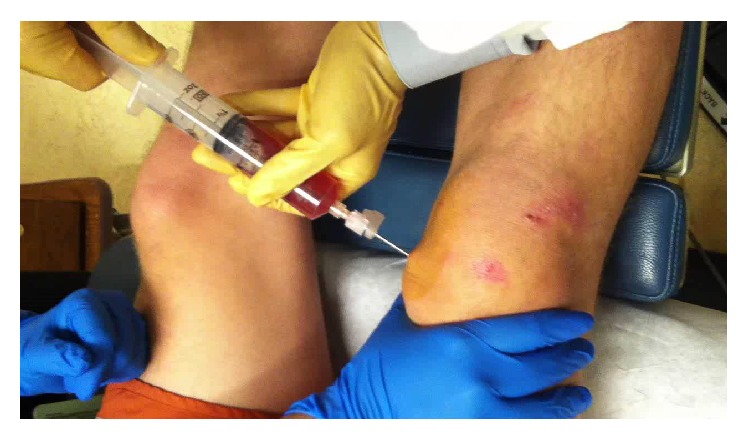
Using ultrasound for needle placement, 38 mL serosanguinous fluid was aspirated.
